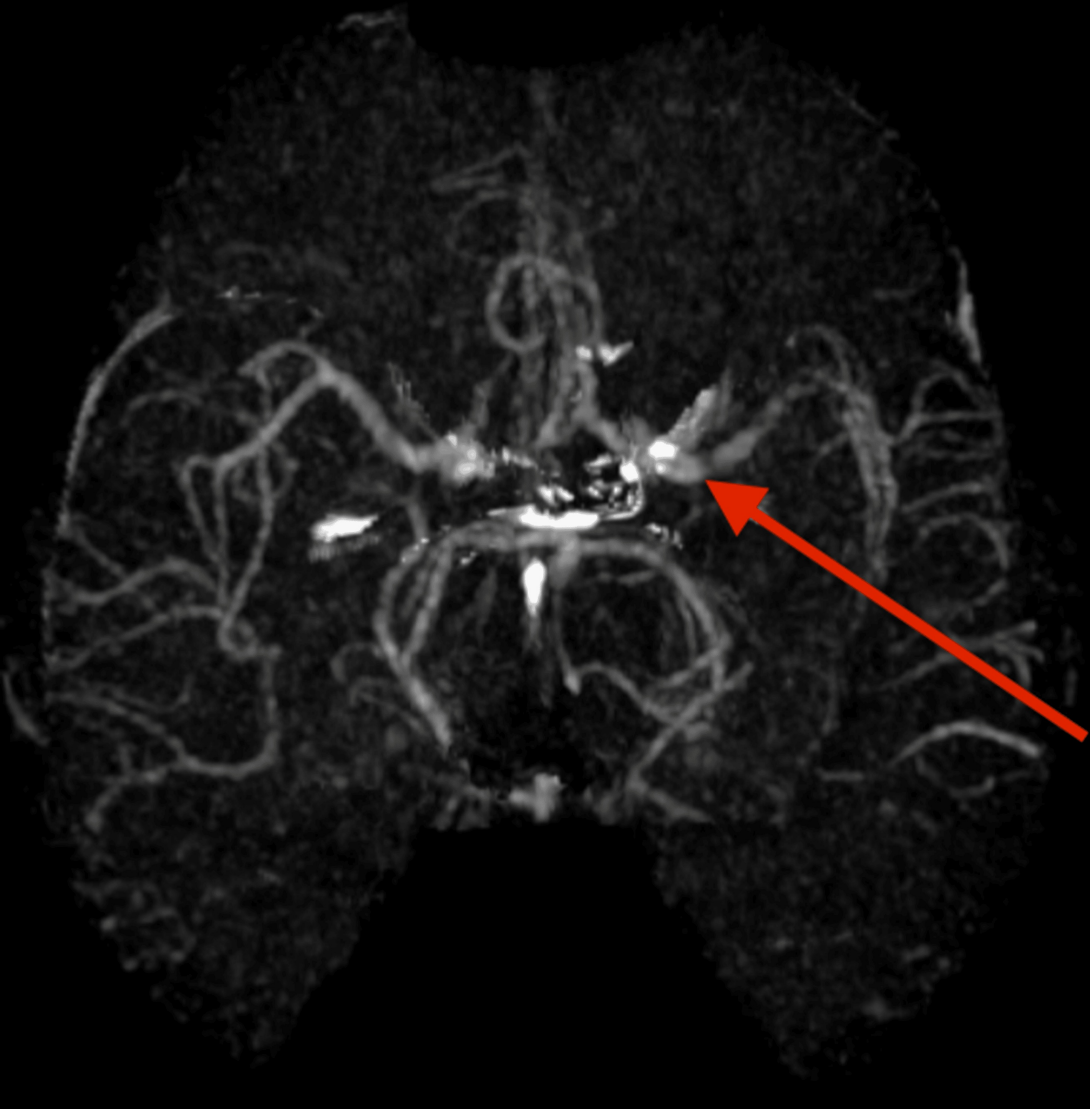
CT Angiography Showing Occlusive Left M1 Middle Cerebral Artery (MCA) Thrombus CT angiogram showing occlusion of the distal left M1 middle cerebral artery (red arrow). Good collateral circulation is seen with retrograde filling of the M2 branches in the left Sylvian fissure.
